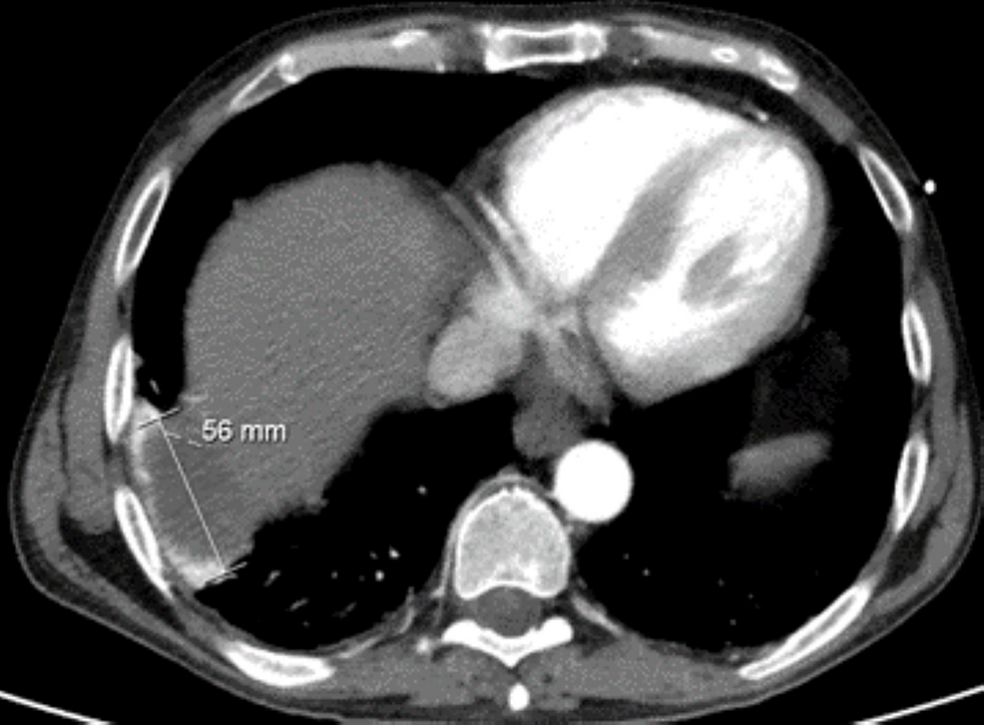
Abdominal CT scan for reassessment following clinical deterioration The dimensions of the hepatic lesion are marked on the figure in millimeters.
